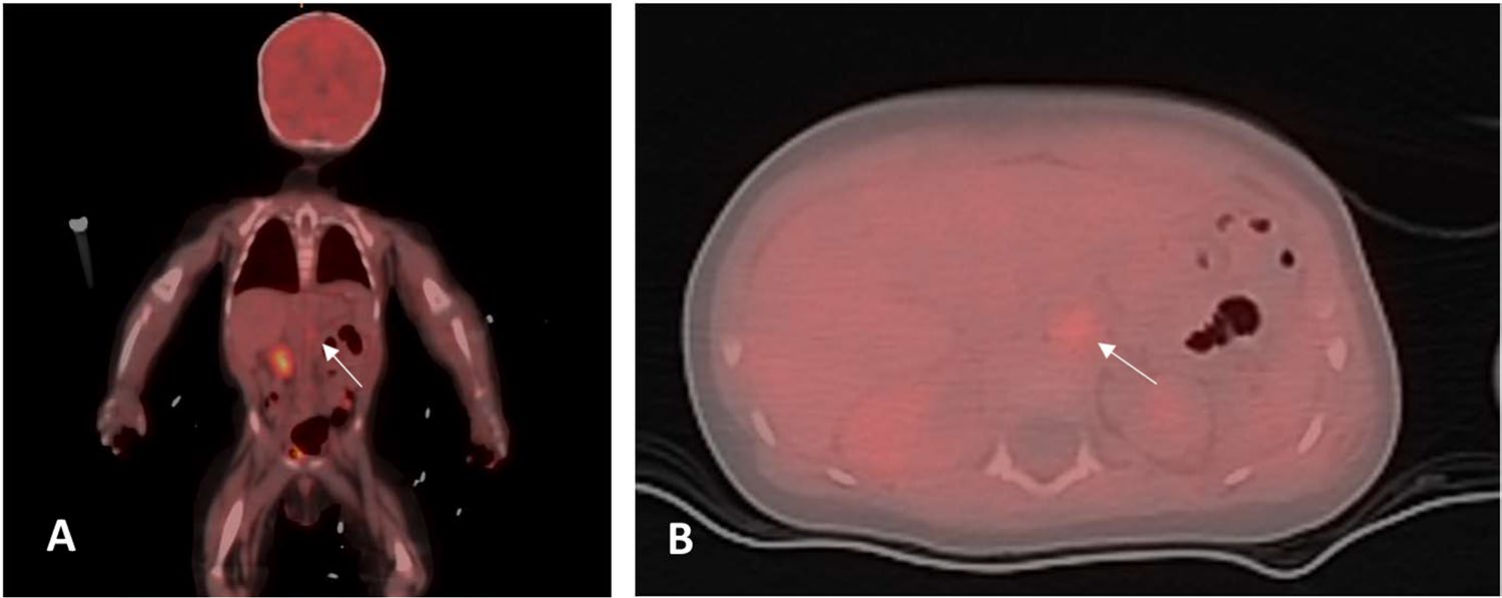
FDG-PET/CT findings at diagnosis. A Coronal view and B axial view demonstrate focal pathological FDG uptake in the abdominal aorta (white arrows), just below the origin of the superior mesenteric artery. This uptake is consistent with active inflammation and supports the diagnosis of large-vessel vasculitis, specifically Takayasu arteritis
